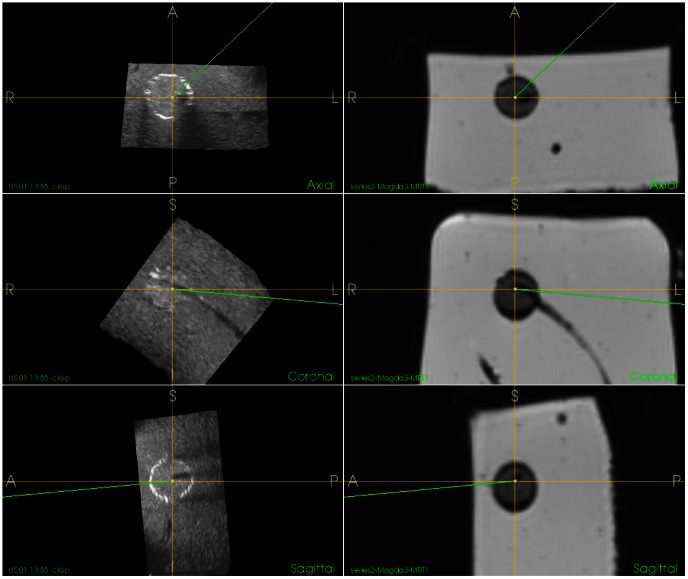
Orthogonal slicing through a 3D ultrasound volume and the MR volume data. From top to bottom: axial, coronal and sagittal slices. The tumor model has a silicone string going into it, representing a portal vein, as can be seen in the coronal slice in both ultrasound and MR.
